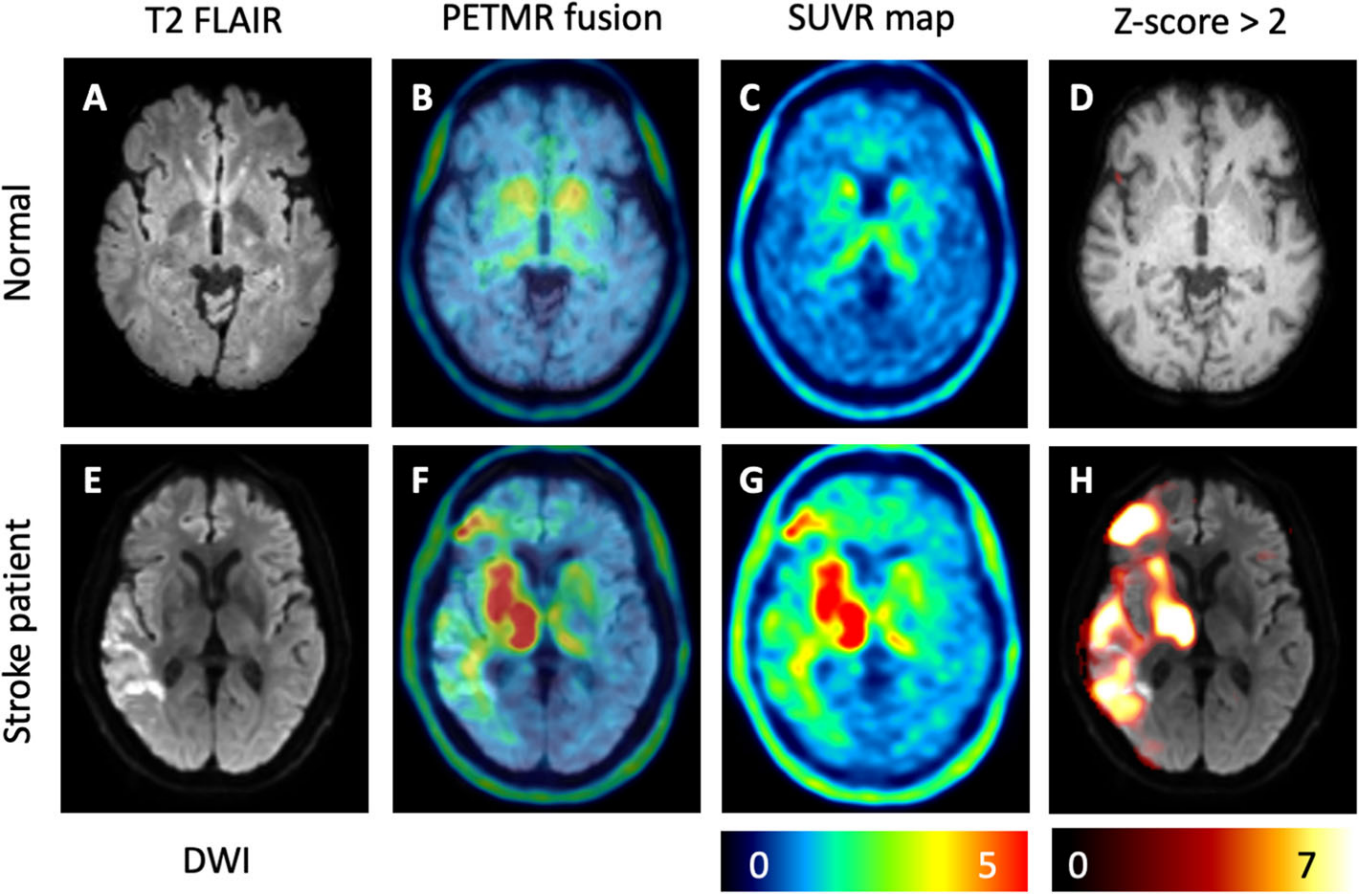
Transforming the ^18^F-THK-5351 SUVR maps to ^18^F-THK-5351 Z-score maps. Regarding a representative healthy subject (a), off-target binding of ^18^F-THK-5351 to the basal ganglia and thalamus was noted on the SUVR maps (b, c). After transforming SUVR maps to Z-score maps, the signals in the basal ganglia and thalamus were robustly diminished (d). Regarding a patient with right hemisphere ischemic stroke (e), ^18^F-THK-5351 uptake signals were increased around the stroke lesion; further, they were asymmetrically elevated in the basal ganglia and thalamus on SUVR maps (f, g). On the Z-score map, the ^18^F-THK-5351 signals on the bilateral basal ganglia and thalamus were markedly suppressed and the stroke-induced ^18^F-THK-5351 uptake was better visualized (h)
